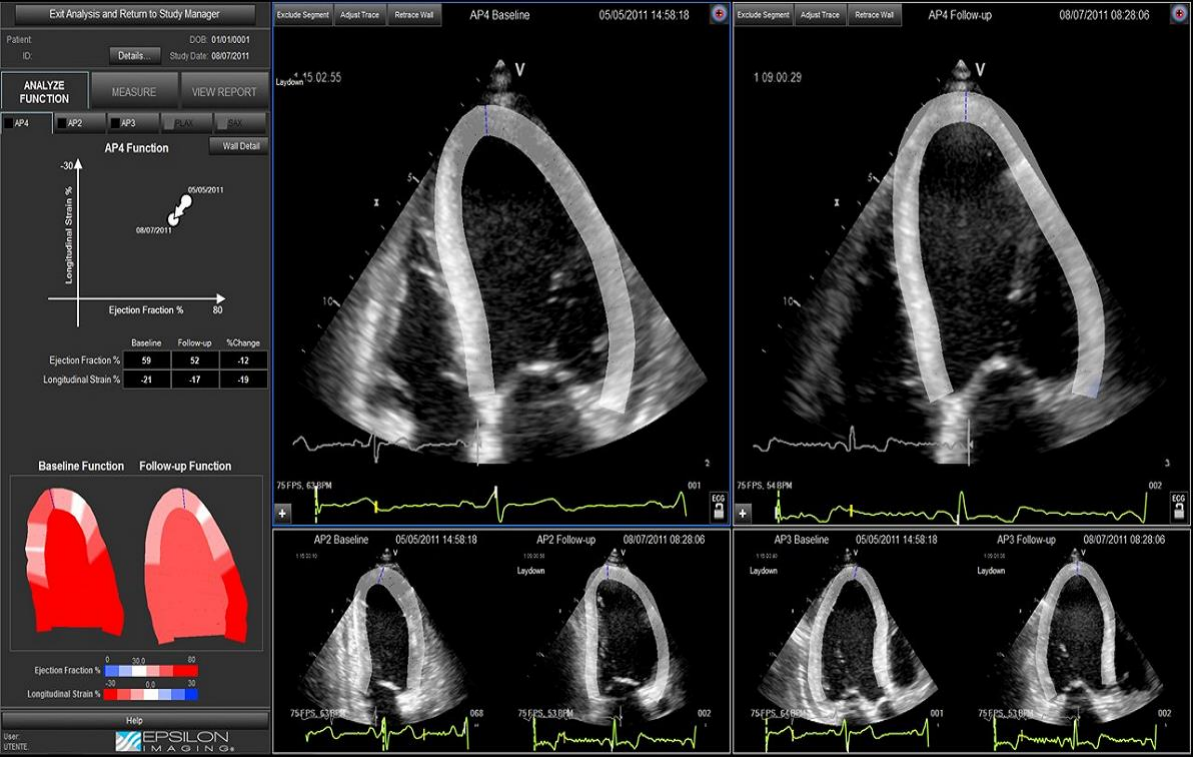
Example of simultaneous baseline and follow up two-dimensional speckle-tracking analysis of the left ventricle (LV) using a vendor independent software and using DICOM images. In this figure the region of interest (ROI) was tracked and left ventricular ejection fraction (LVEF) and LV global longitudinal strain (LVGLS) were computed: A. Baseline analysis of apical four chambers view; B. Follow-up analysis of apical four chambers view; C. Baseline analysis of apical two chambers view; D. Follow-up analysis of apical two chambers view; E. Baseline analysis of apical three chambers view; F. Follow-up analysis of apical three chambers view; G. Integrated display of changes in LVEF and LVGLS from baseline to follow-up; H. LVEF and LVGLS baseline and follow up values and changes as proportions of the initial value. I. Baseline and follow up colored map of the ventricle wall.
